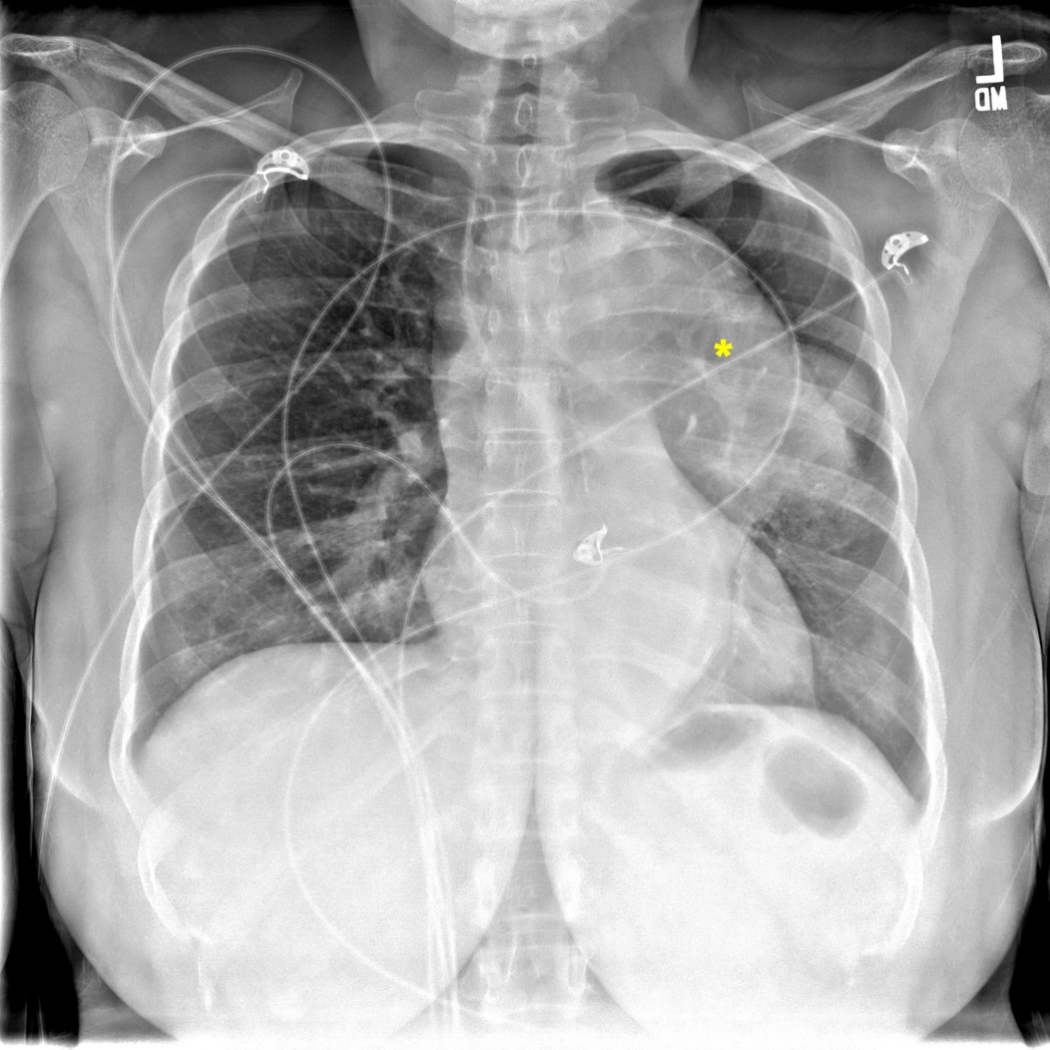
Anteroposterior view chest X-ray showing a mediastinal lesion (*).
